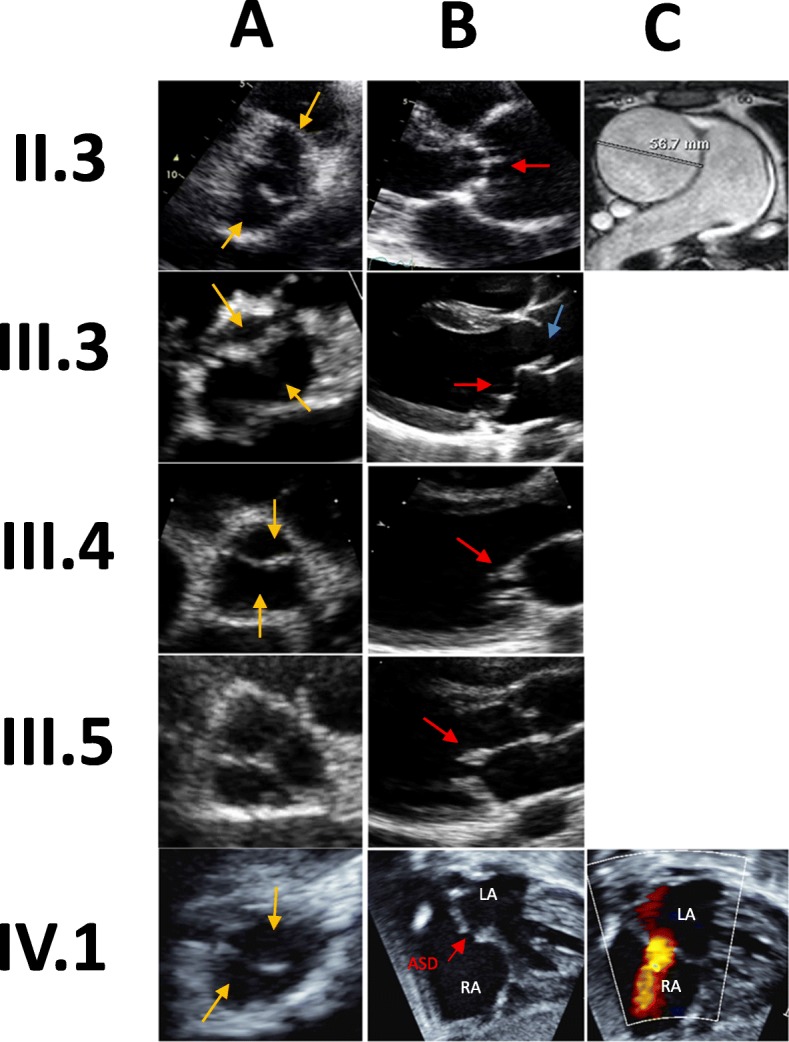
Echocardiogram findings for previously reported family members. Legend: II.3 A: Anterior and posterior aortic leaflets (orange arrows) of a bicuspid aortic valve. II.3B: Calcified aortic valve (red arrow) with a dilated ascending aorta. II.3C: Cardiac MR image showing the ascending aortic aneurysm dilated at 5.8 cm. III.3A: Two leaflets of the bicuspid aortic valve (orange arrows). III.3B: Doming of the aortic valve (blue arrow), consistent with a bicuspid aortic valve, and mitral valve prolapse (red arrow). III.4A: Anterior and posterior leaflets (orange arrows) of a bicuspid aortic valve. III.4B: Thickened mitral valve (red arrow). III.5A: Tri-leaflet aortic valve. III.5B: Thickened mitral valve leaflets (red arrow). IV.1A: Bicuspid aortic valve leaflets (orange arrows). IV.1B-C: Septal defect between the left and right atrium
